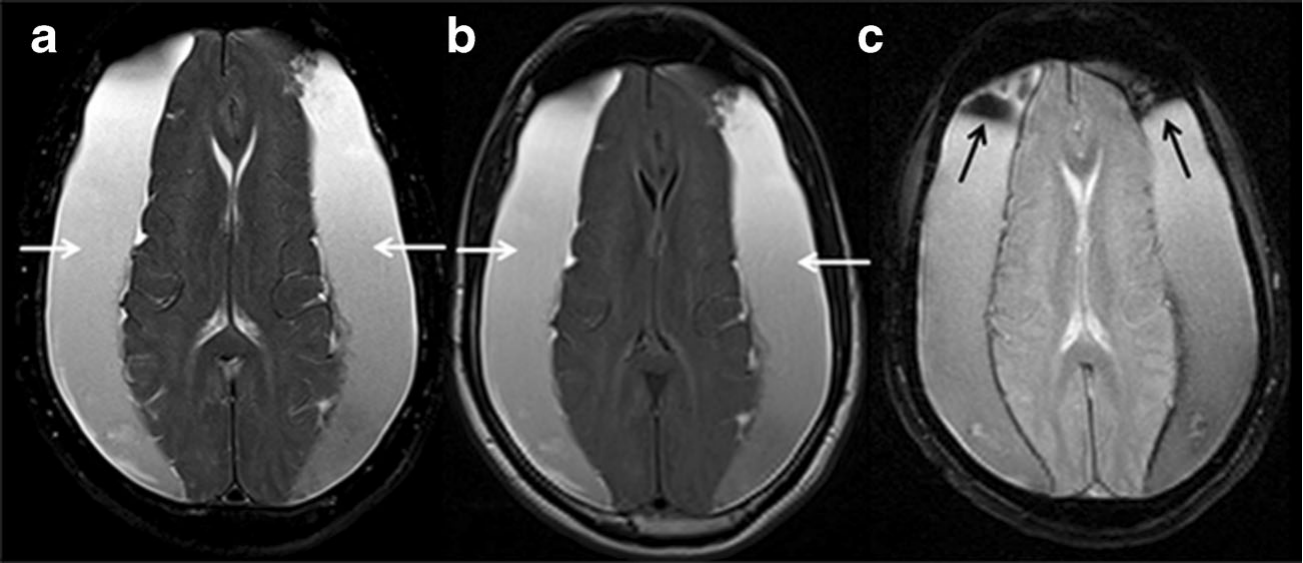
Chronic subdural hematoma (SDH). A 73-year-old male with trivial head trauma later developed progressive loss of cognitive function. a & b axial T2W and FLAIR sections of brain show hyperintense extra axial fluid collection in bilateral cerebral convexities (*white arrows*) with severe compression of adjacent cerebral hemispheres and effacement of the ventricles & sulci. c axial GRE image of the brain demonstrate predominantly hyperintense fluid in bilateral cerebral convexities with hypointense margin on the medial aspect. There are also localized hypointensities (blooming susceptibility artefacts from the blood products) on the anterior aspects of the collections (*black arrows*)
